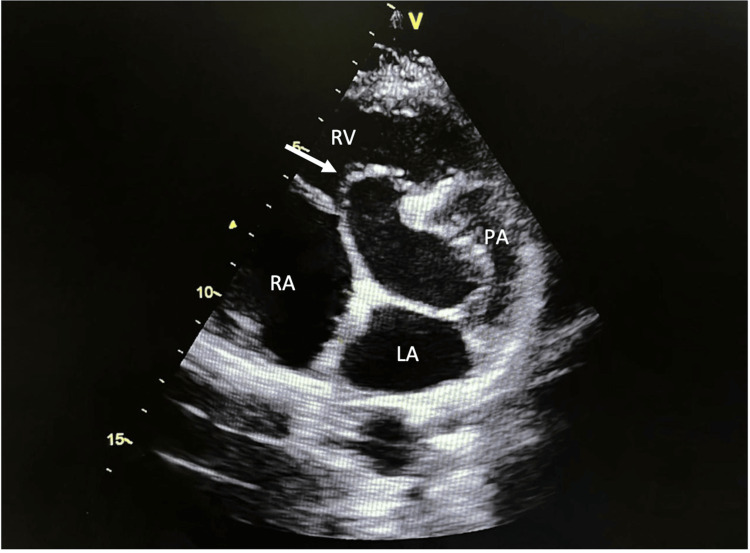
Modified transthoracic parasternal short-axis view showing the membranous interventricular septal aneurysm (arrow). LA: left atrium; PA: pulmonary artery; RA: right atrium; RV: right ventricle.
